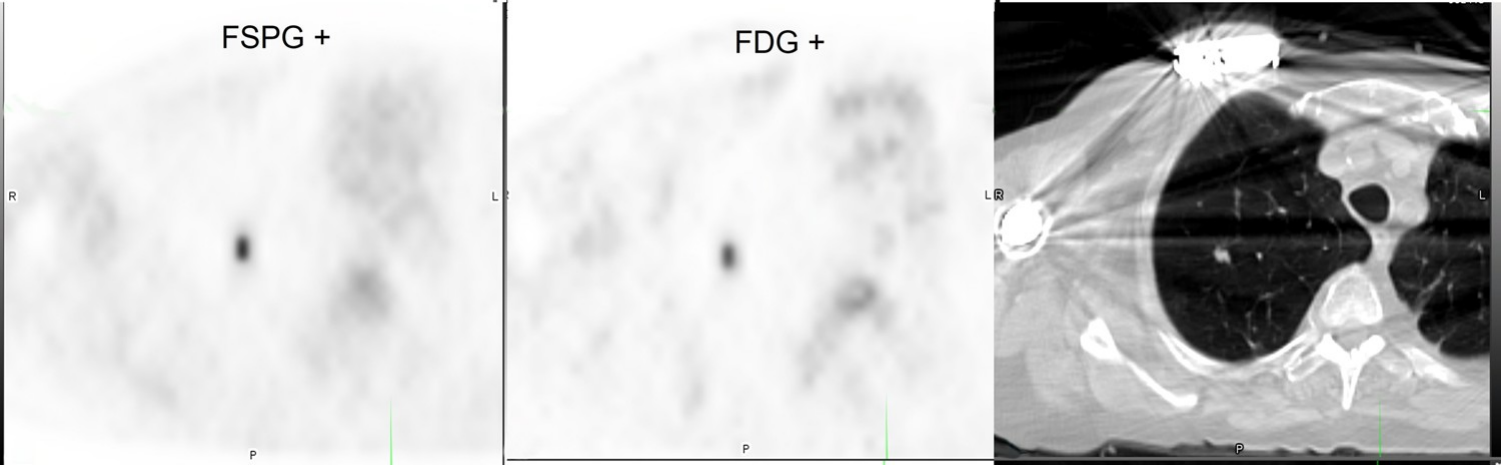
Subject with positive ^18^F-FSPG (SUVmax 3.5) and ^18^F-FDG (SUVmax 7.4) PET/CT.
